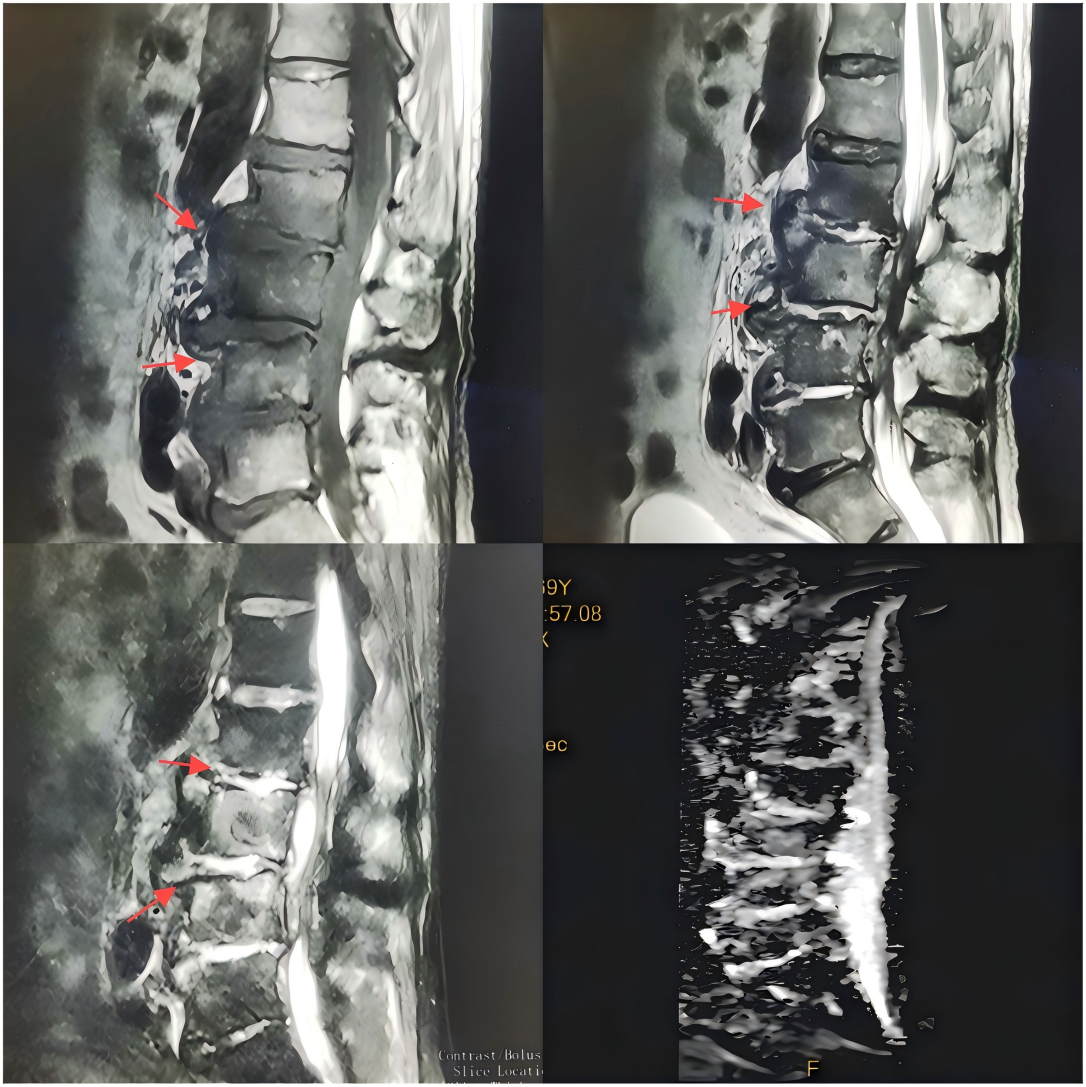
T1-weighted MR images of the intervertebral discs acquired with varying flip angles.
